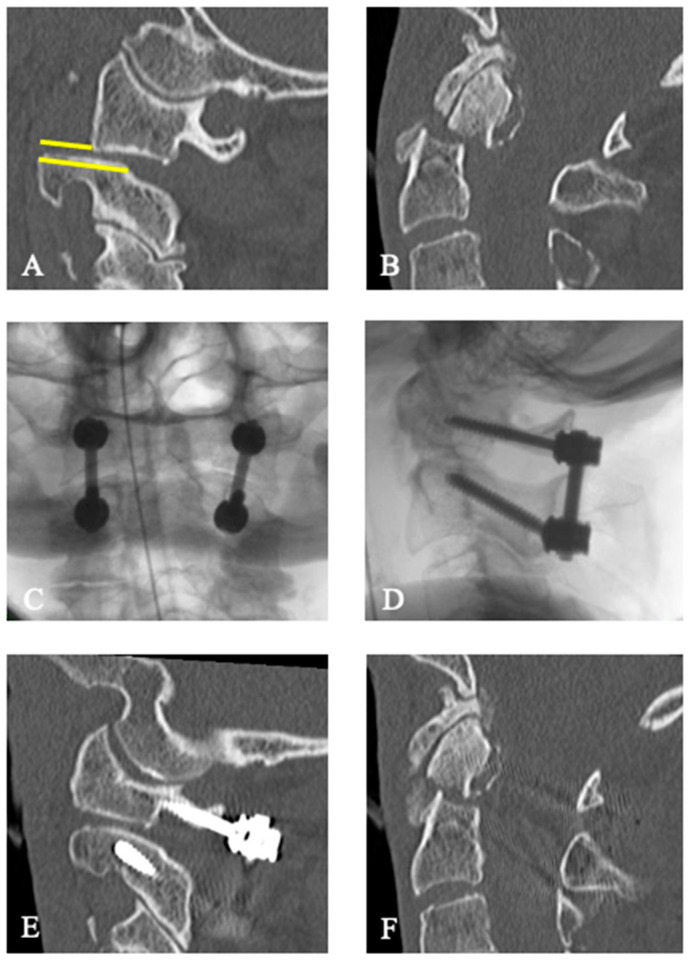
73 year old male patient with a type II odontoid fracture, according to Anderson and D’Alonzo and AAI (Case 2). The C2-uncovering is > 50% ((A), yellow line), and dens’ apex is dislocated (B). Intraoperative fluoroscopy of the dorsal atlantoaxial instrumentation in lateral (C) and anterio-posterior views. (D). Postoperative computed tomography with the correct position of the lateral C1/C2 joint (E) and reposition of the dens’ apex (F).
